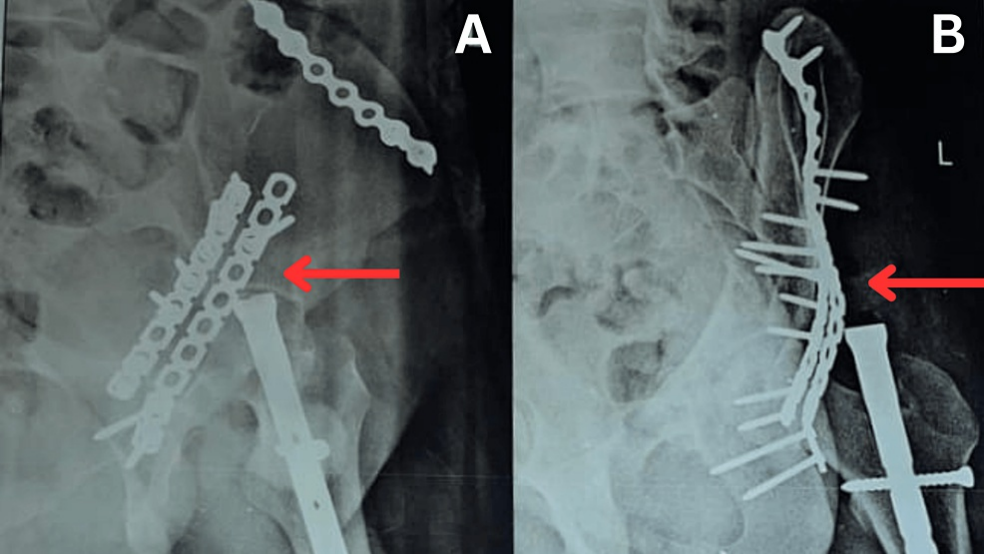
X-ray showing post-operative anterior column PF of left side Figure 1A: Arrow shows anterior ring stabilisation done with plates and screws (anterior-posterior view) Figure 1B: Arrow shows anterior ring stabilisation done with plates and screws (lateral view) PF: Pelvic fracture
